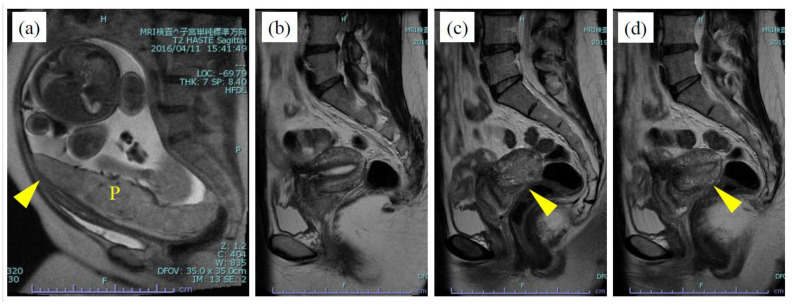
Sagittal T2-weighted MR images of Case 13. They showed placenta previa at the cranioventrally stretched internal cervical ostium (arrowhead) at 33 weeks of gestation (a). MRI after the delivery showed signs of severe adhesion (b); retroflexed uterus (c); elevated posterior vaginal fornix (d); loss of the fatty layer at the posterior cul-de-sac between the posterior uterine wall and the sigmoid colon (b–d). P, placenta. MRI imaging of the uterus without using contrast agent.
